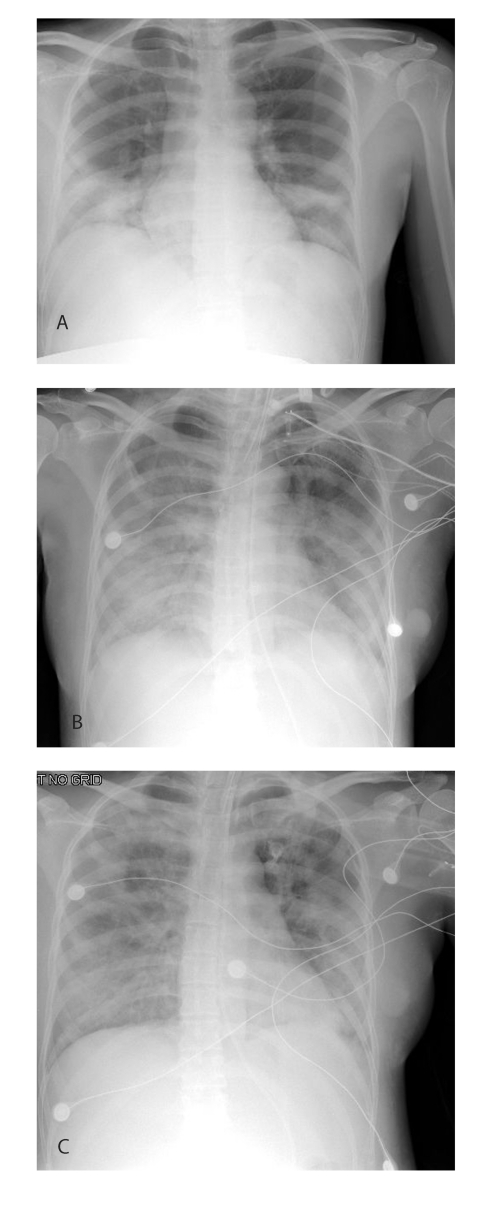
Chest radiographs of case-patient with severe acute respiratory syndrome (SARS) while pregnant. a, day 6 of illness; b, day 10; c, day 13.
